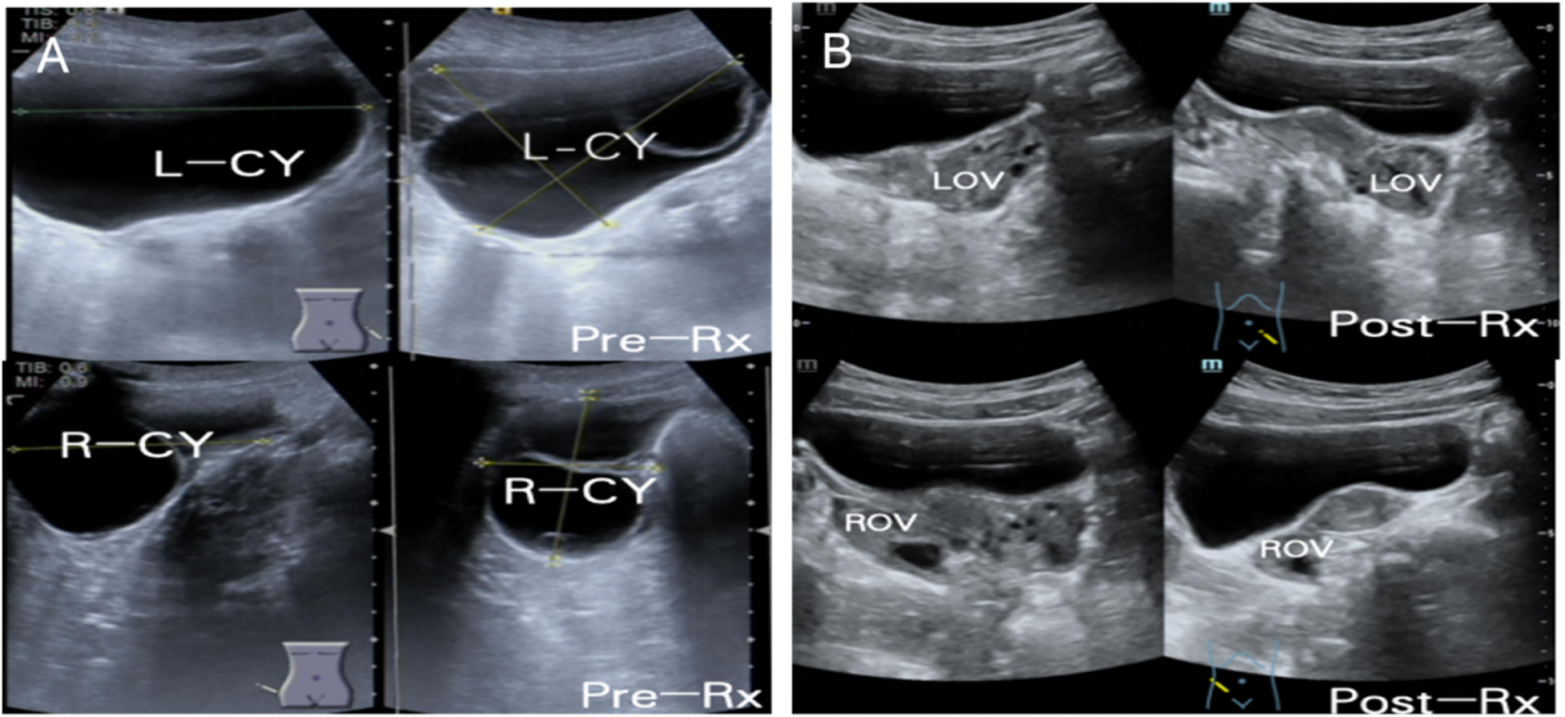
Transabdominal gynecological ultrasound before and after therapy. (A) Pre-treatment scan showing bilateral polycystic ovarian cysts (right: 61 × 61 × 42 mm; left: 98 × 93 × 71 mm) with residual ovarian tissue visible peripherally. (B) Follow-up scan obtained 4 weeks after levothyroxine therapy showing marked reduction of cystic lesions and return to normal ovarian morphology.
